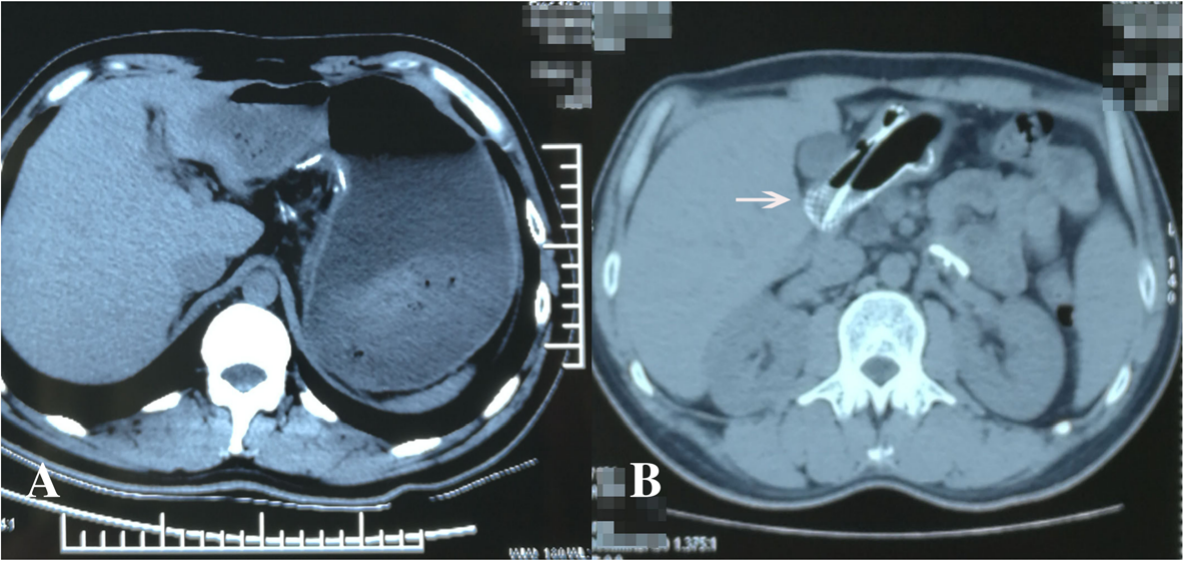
CT of the abdomen. a Gastric dilatation 12 h after a meal on the 3rd postoperative day. b Placement of a SEMC 4 months after surgery (→: SEMC)
